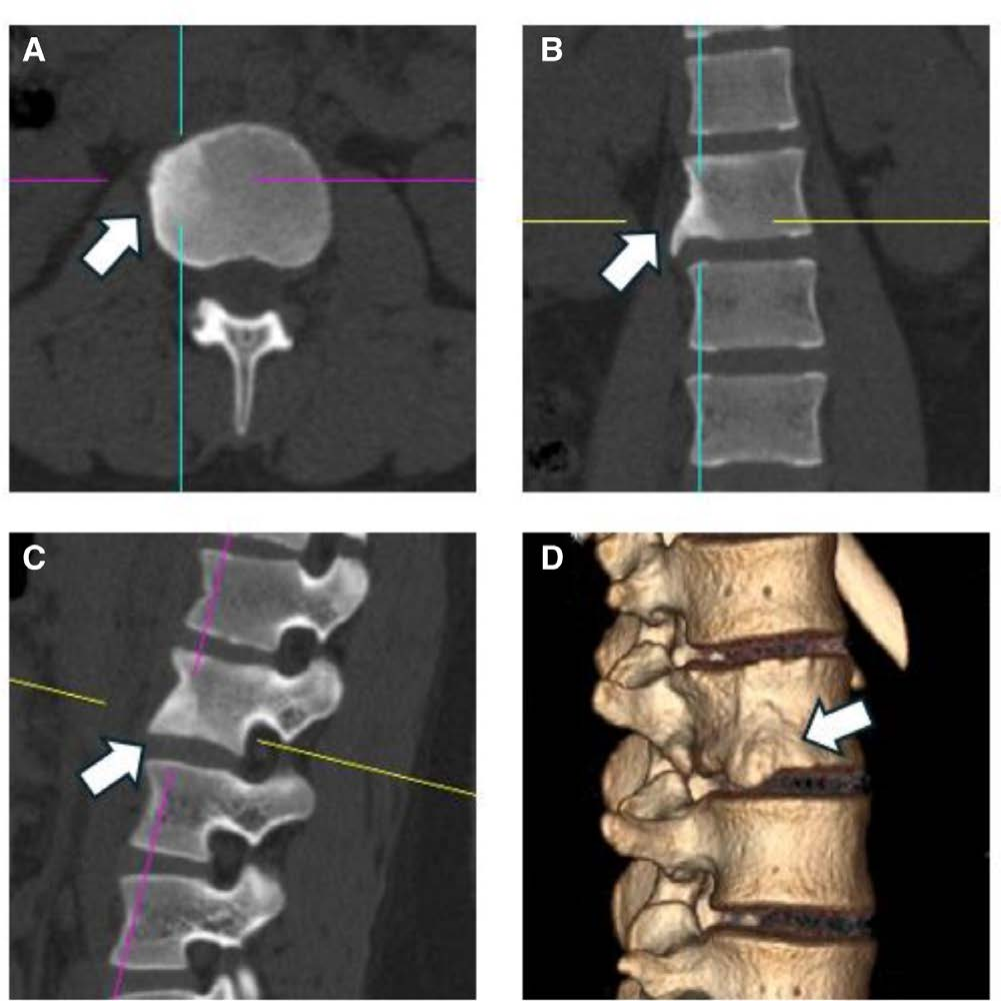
CT images obtained after discharge nearly 1.5 yr. Horizontal (A), sagittal (B), and coronal (C) CT images and 3D reconstruction (D) show that bone sclerosis has formed at the site of the lesion. CT = computed tomography.
